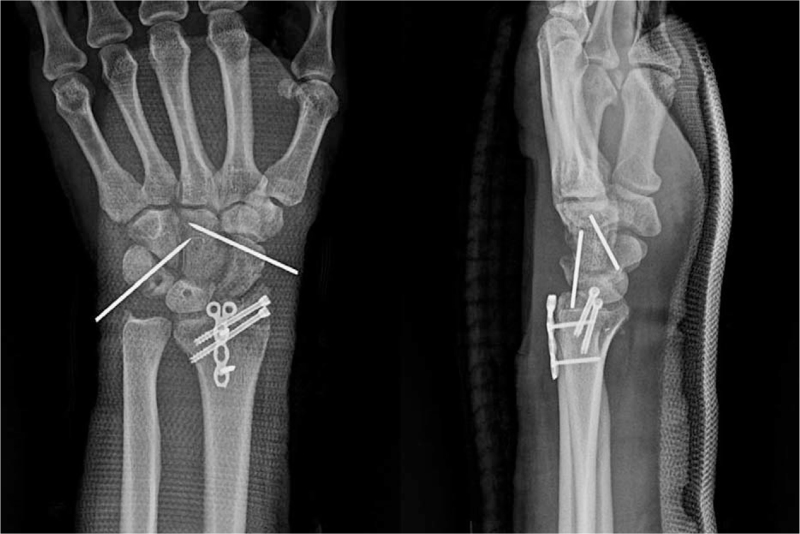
Postoperative radiographs demonstrate a well-reduced fracture fragments and carpal alignment.
